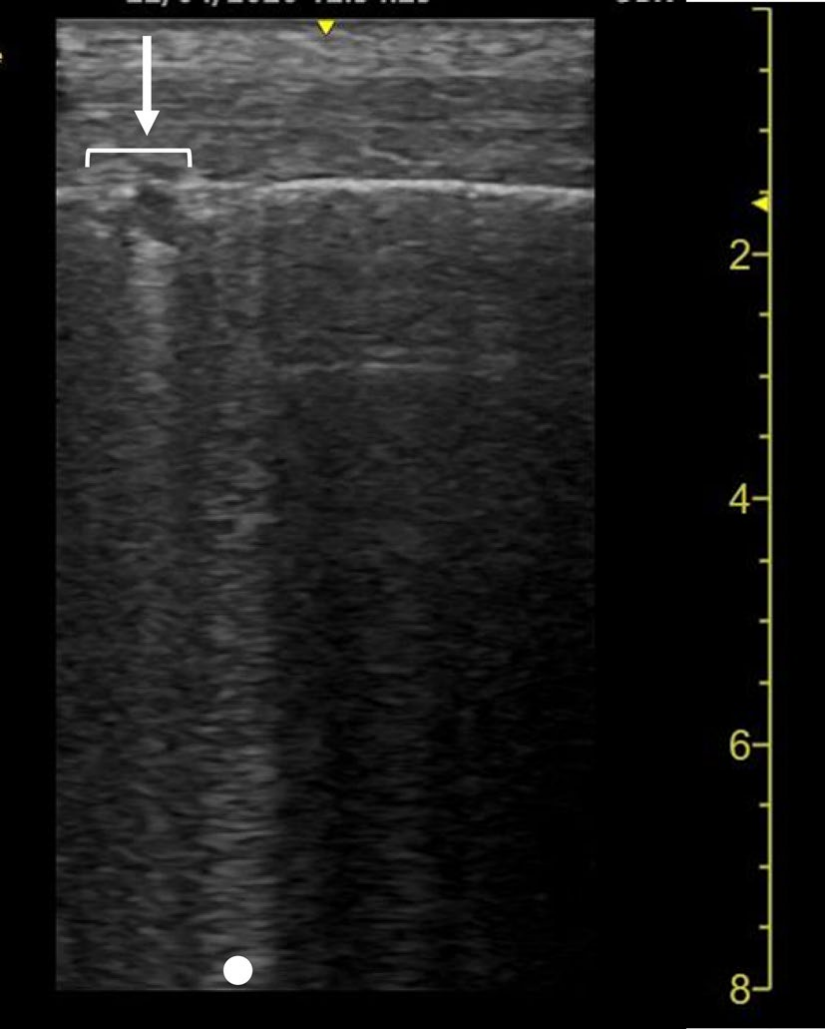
A lung ultrasound image of community-acquired pneumonia. A small subpleural consolidation (arrow) is shown with a B-line (dot). A normal sign is shown on the right side of the image
